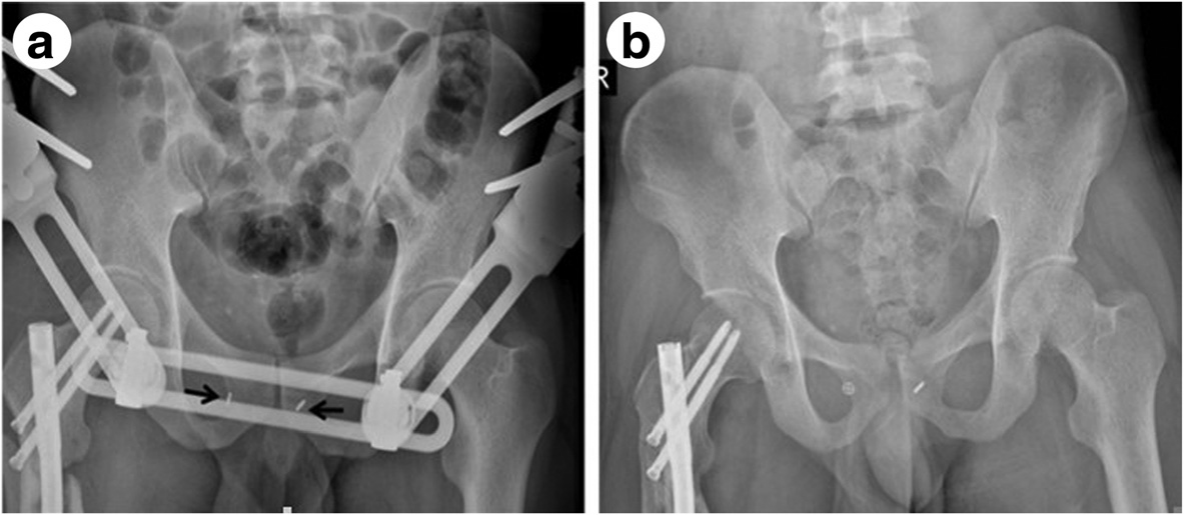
Postoperative radiographs. a Immediately after surgery. b One year after surgery
